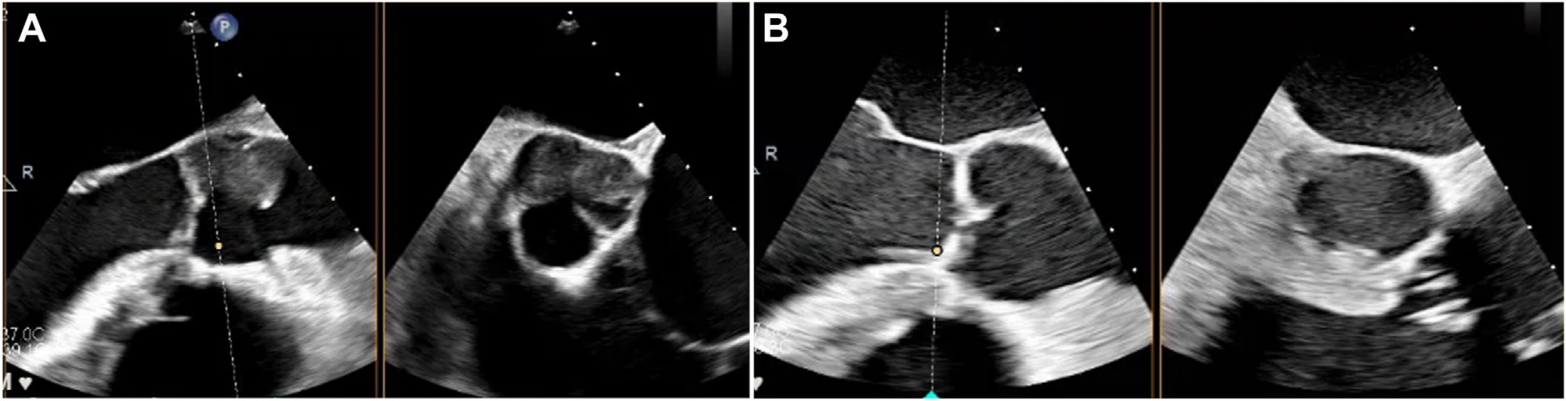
Intraoperative transesophageal echocardiography demonstrating (A) preoperative 2.3 × 2.3-cm thrombus in aortic root along left and noncoronary cusps of the aortic valve with evidence of left coronary artery occlusion and (B) after successful thrombectomy.
